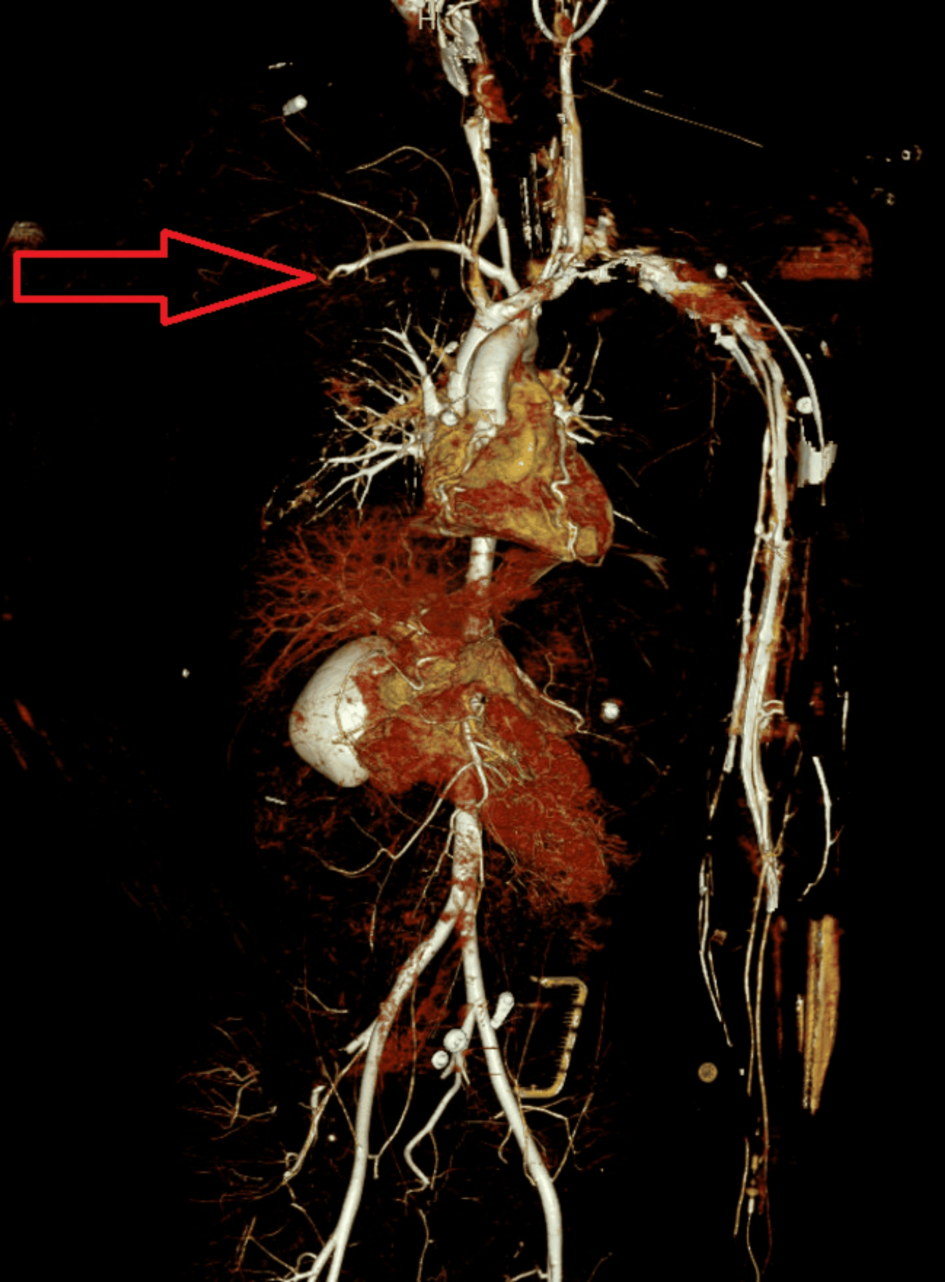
CT angiogram view demonstrating lack of blood supply in the right upper limb The red arrow demonstrates that there is lack of blood supply in the right upper limb as compared to the left upper limb.
